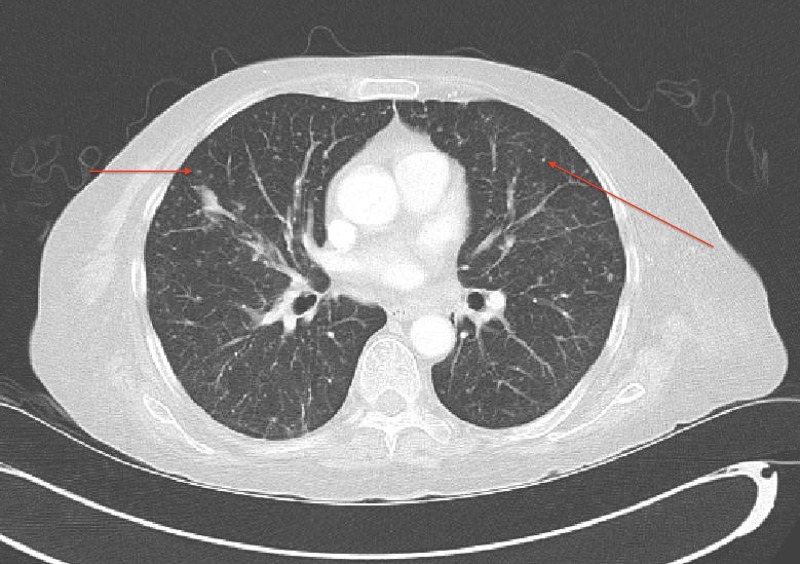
Computed tomography scan of the chest showing multiple small, bilateral pulmonary nodules (red arrows) consistent with miliary tuberculosis
